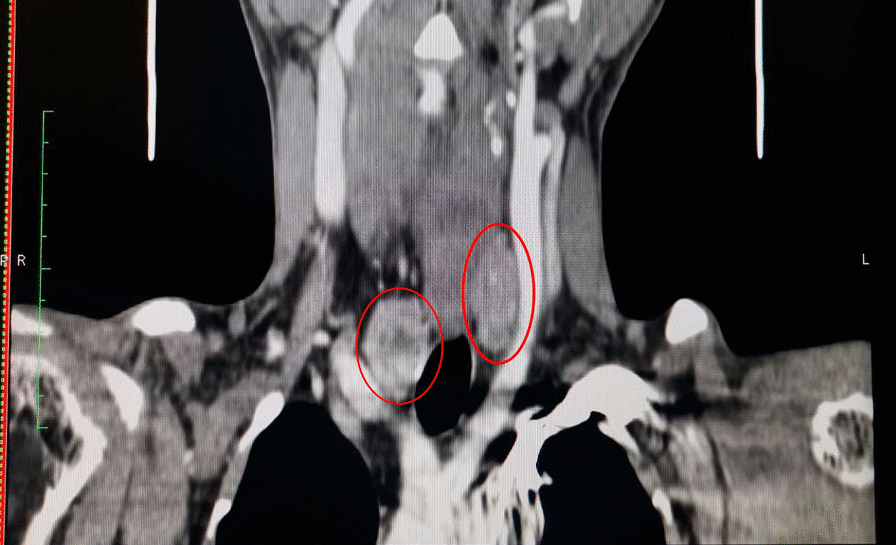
Computed tomography of the neck with IV contrast. Bilateral heterogeneous nodules in the region of the parathyroid glands (red ovals)
